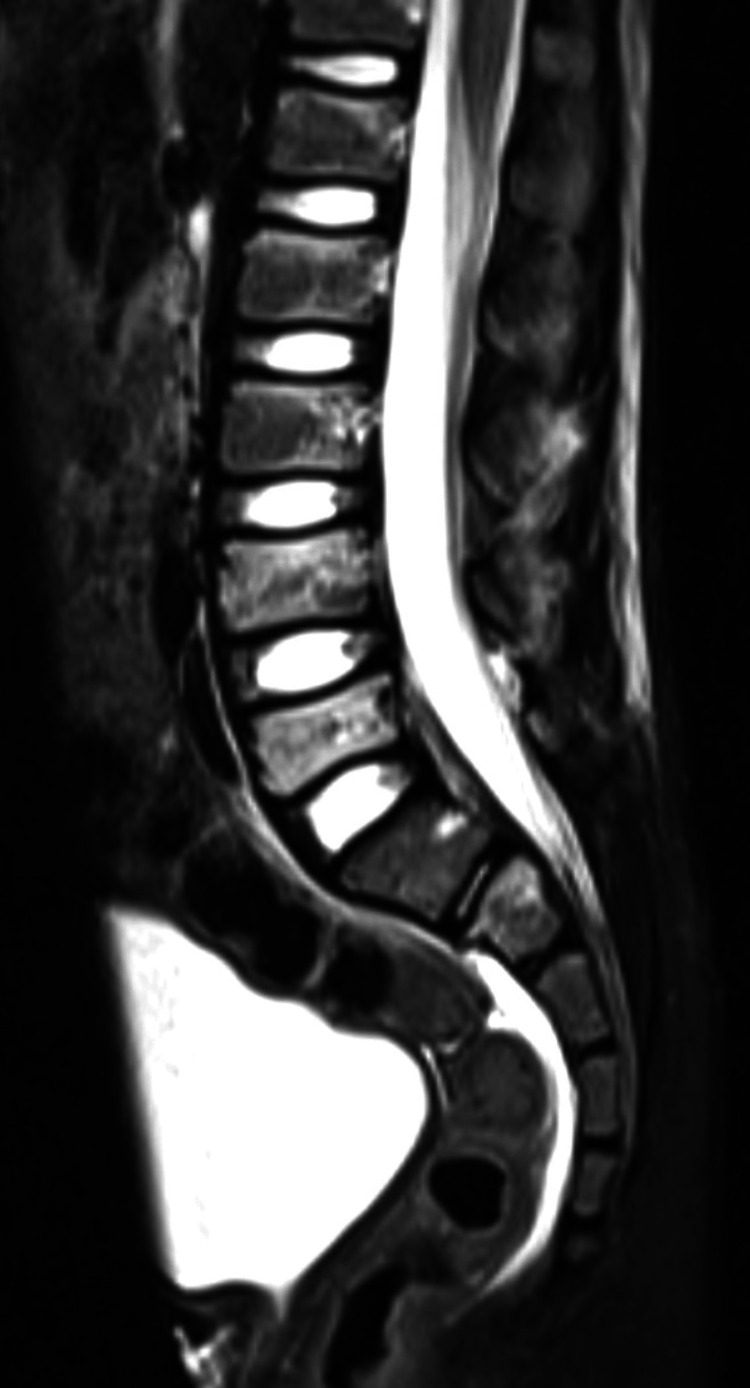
MRI showing injuries of the yellow ligaments and absence of disc injuries
